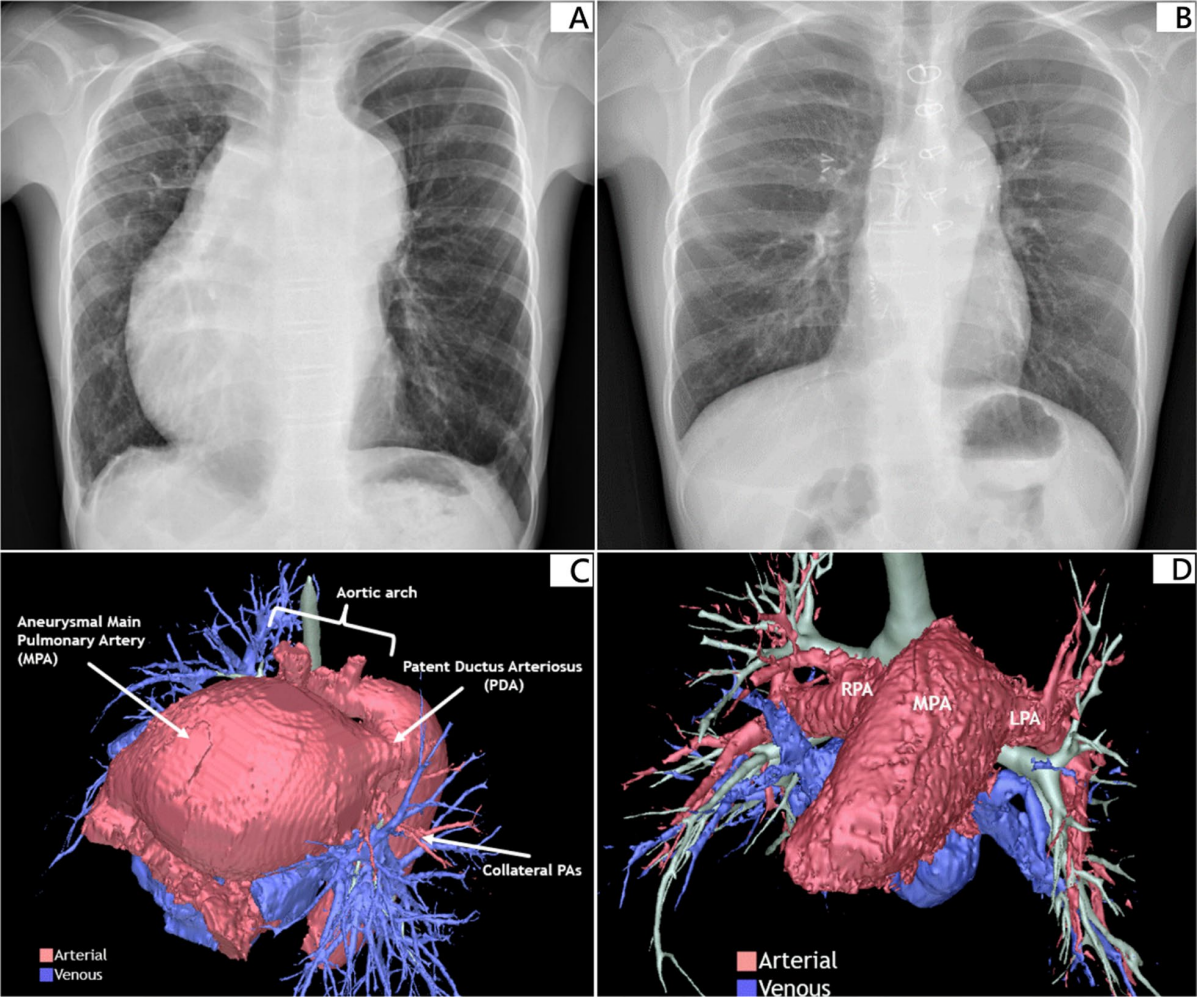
Chest radiograph (CXR) and three-dimensional reconstructed chest computed tomography (3DrCT) before and after lung transplantation. A CXR shows engorged main pulmonary artery and right-side cardiomegaly before lung transplantation. B CXR shows normal heart size with good expansion of the bilateral lung field one year after lung transplantation. C Preoperative 3DrCT provides clear visualization of the cardiac anatomy. Hypoplasia of the bilateral pulmonary artery with some small collateral vessels from systemic circulation (pink color), aneurysmal dilatation of the main pulmonary trunk, and a patent ductus arteriosus are well demonstrated in the reconstructive photography. D Postoperative 3DrCT shows the virtual images of bronchial trees and the pulmonary vessels 11 months after transplantation (pink color indicates pulmonary arterial system and blue color shows pulmonary venous system)
